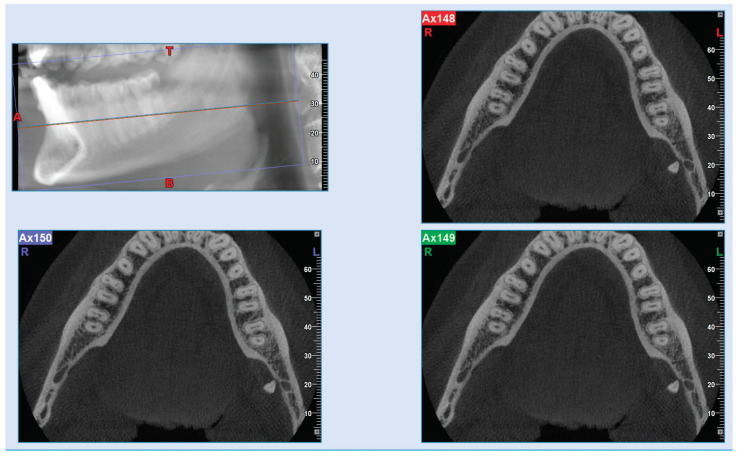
CBCT sagittal and axial views showing the position of the residual fractured root outside the bone in the submandibular space.
